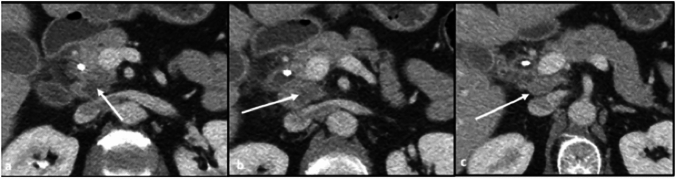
Axial CT sections with portal venous phase contrast. a, b Hypoenhancing mass in the posterior pancreatic head/uncincate process (arrows). c Abnormally enlarged, hypoenhancing retropancreatic node, indicating malignant involvement (arrow)
